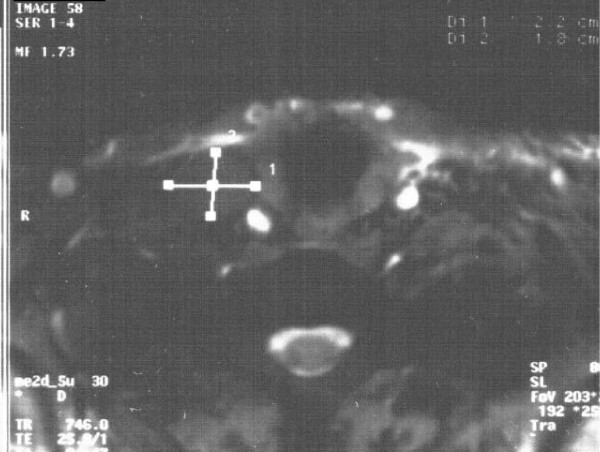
Contrast-enhanced CT of the chest demonstrating thrombosis at the level of the right subclavicular vein.
